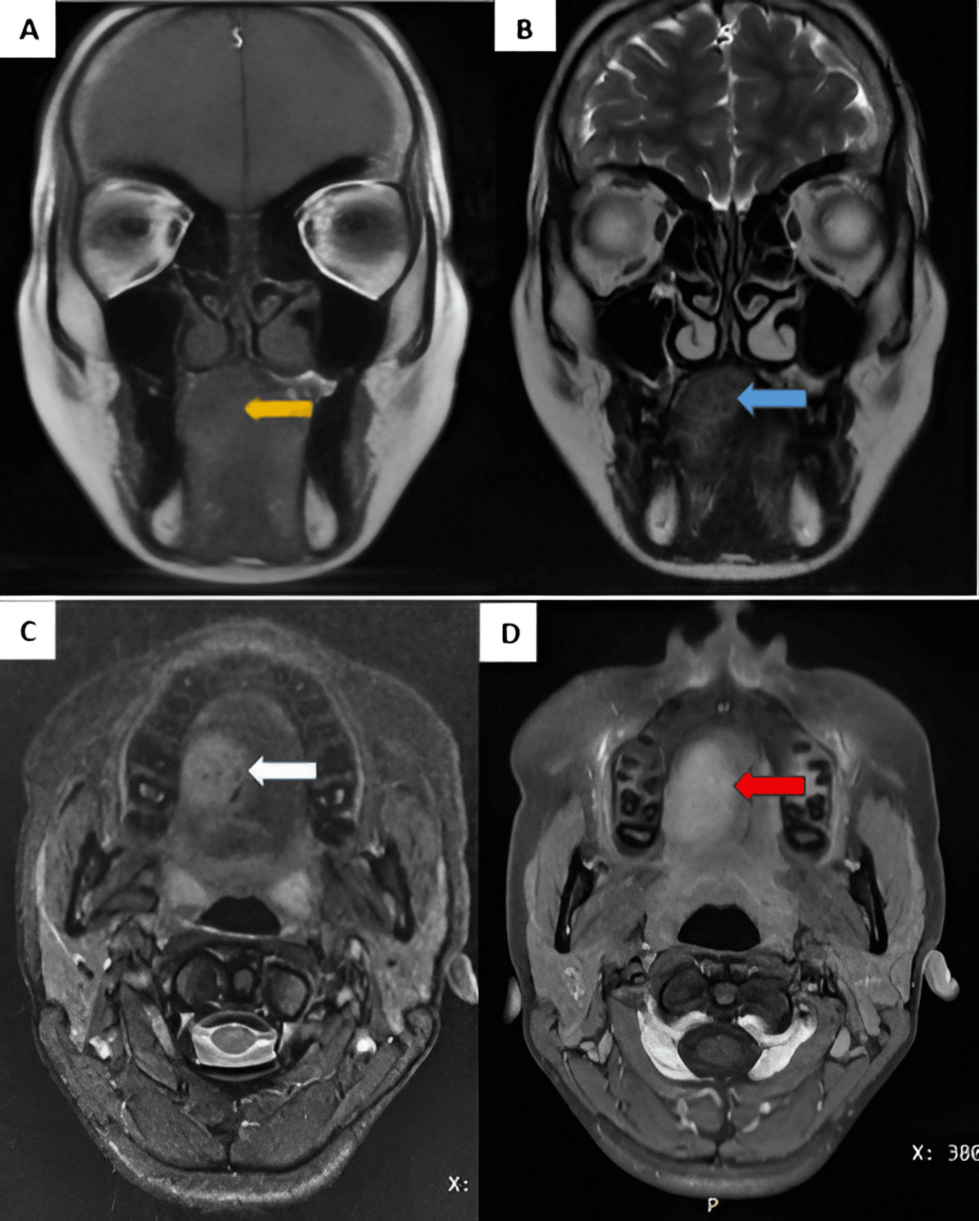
(A) Coronal T1WI, (B) coronal T2WI, (C) axial STIR, and (D) axial T1 + C A well-defined altered signal intensity lesion on the dorsal aspect of the tongue on the right side appearing T1WI isointense (yellow arrow) as compared to the muscle and T2WI (blue arrow)/STIR hyperintense, containing flow voids (white arrow), and showing homogeneous post-contrast enhancement (red arrow) T1WI: T1-weighted image, T2WI: T2-weighted image, STIR: short-tau inversion recovery, T1 + C: post-contrast T1-weighted image
